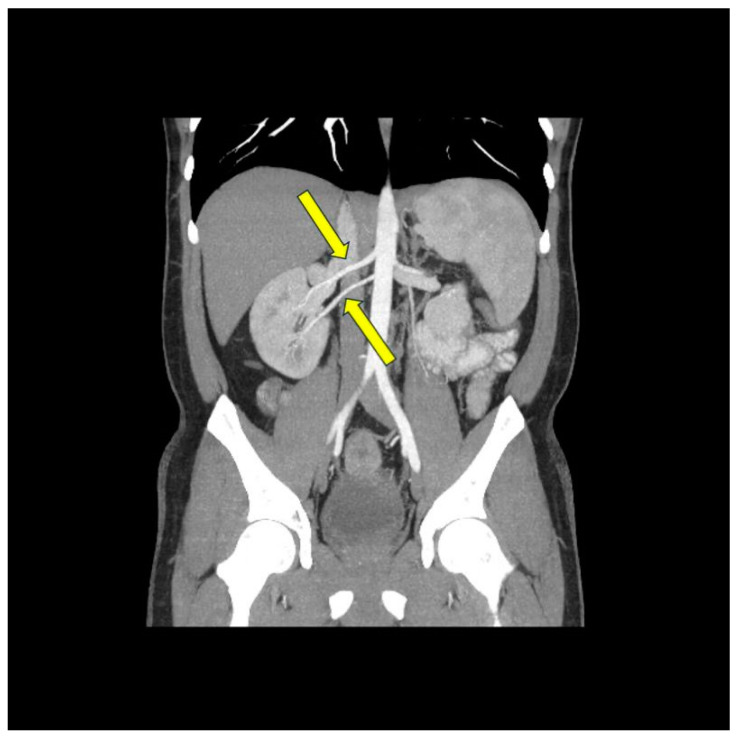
Contrast-enhanced computed tomography scan showing multiple right renal arteries (yellow arrows).
